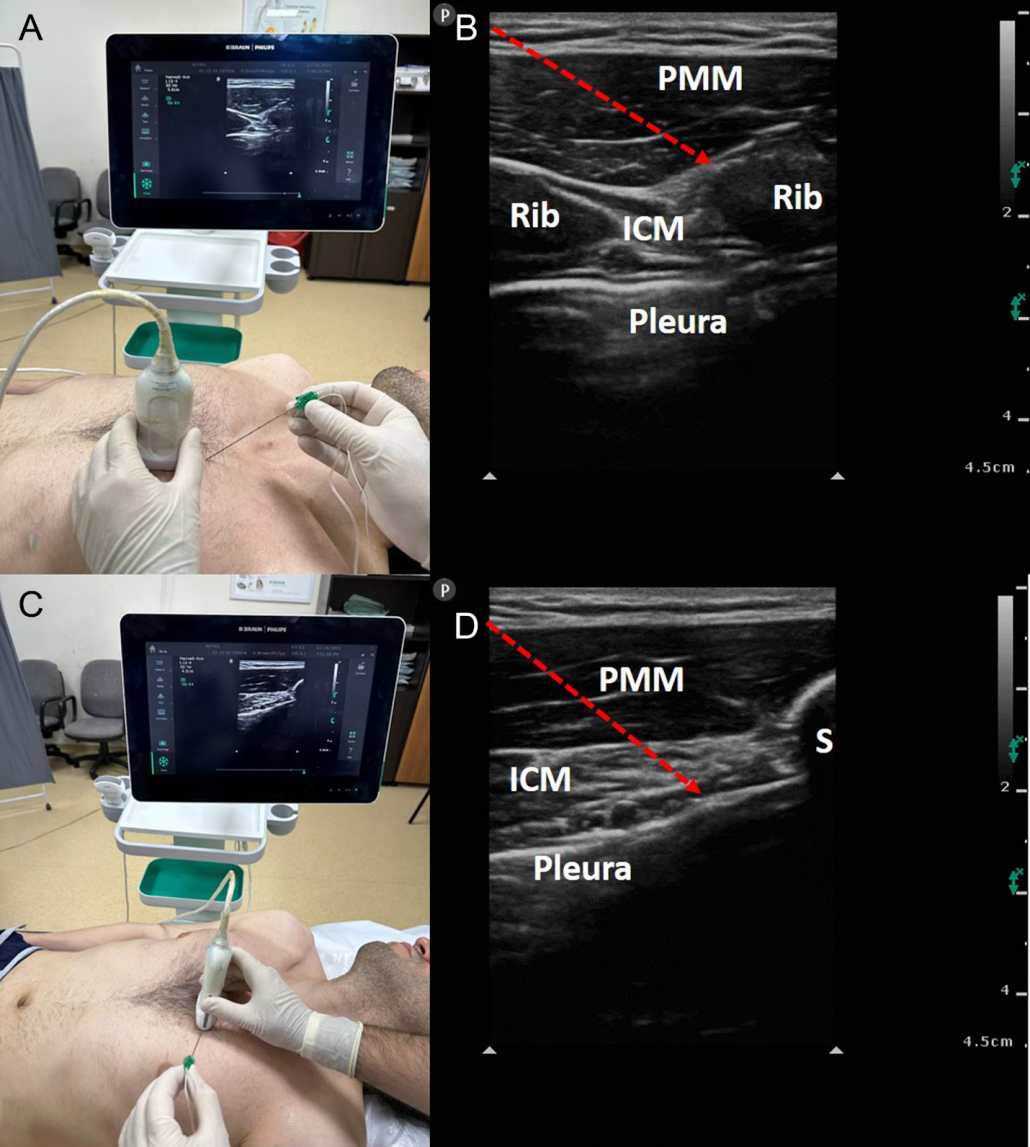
Patient and ultrasound probe position for superficial (A) and deep (C) parasternal intercostal plane blocks procedure. (B, D) Sonographic anatomy of the block. ICM, intercostal muscles; PMM, pectoralis major muscle;red arrow, needle; S, sternum.
